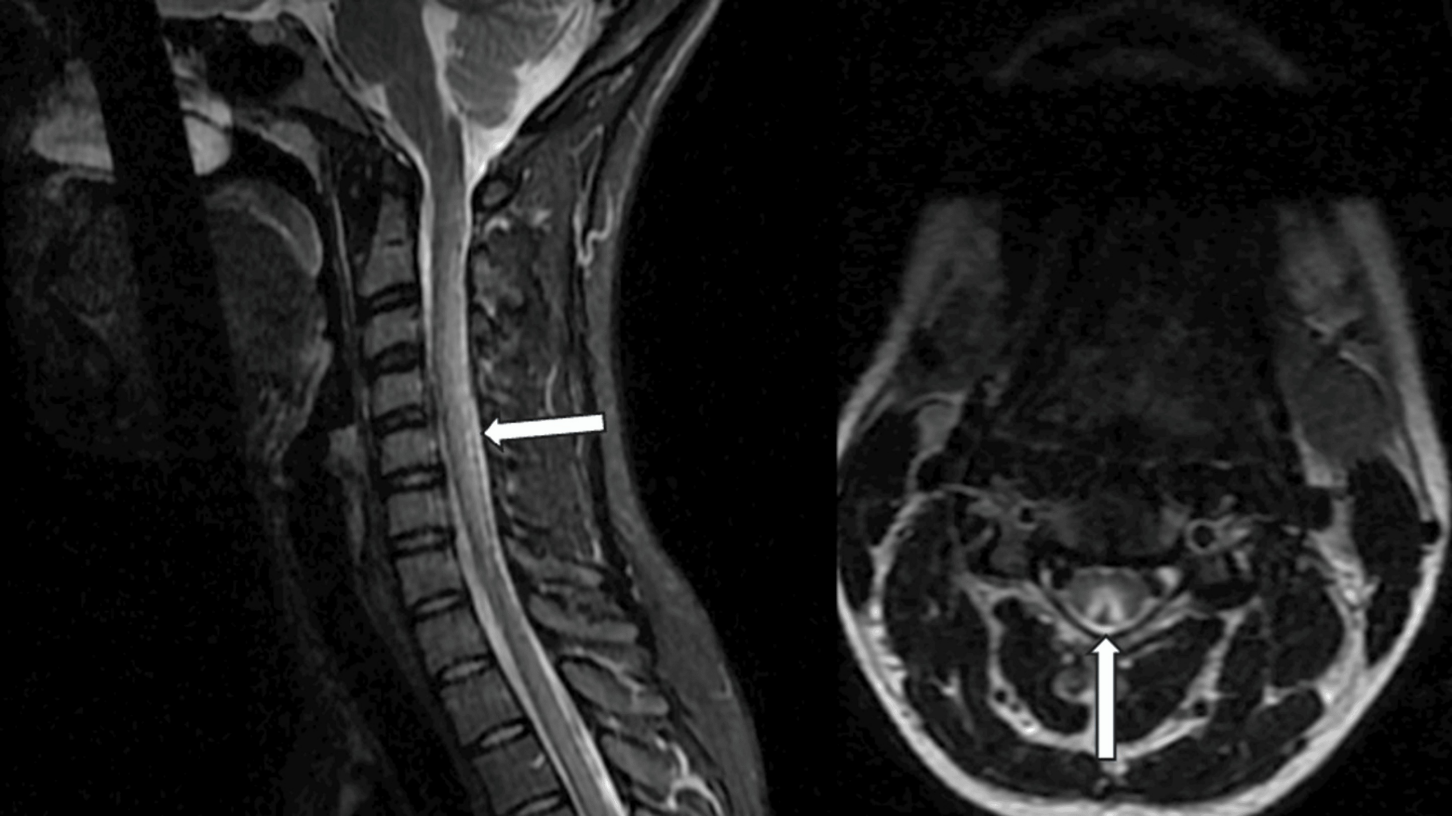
Nitrous oxide-induced myelopathy. T2-weighted hyperintensities are observed longitudinally in the dorsal column of the cervical spinal cord, sagittal view (left), and axial view (right) with an 'inverted V' sign.
